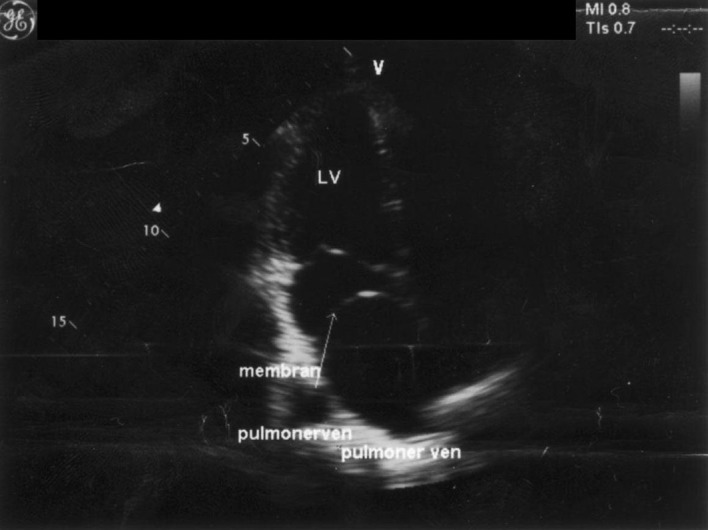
Apical four-chamber echocardiographic images. In the left atrium (LA), the fibromuscular septum is visible. LV: left ventricle; RA: right atrium; RV: right ventricle.
